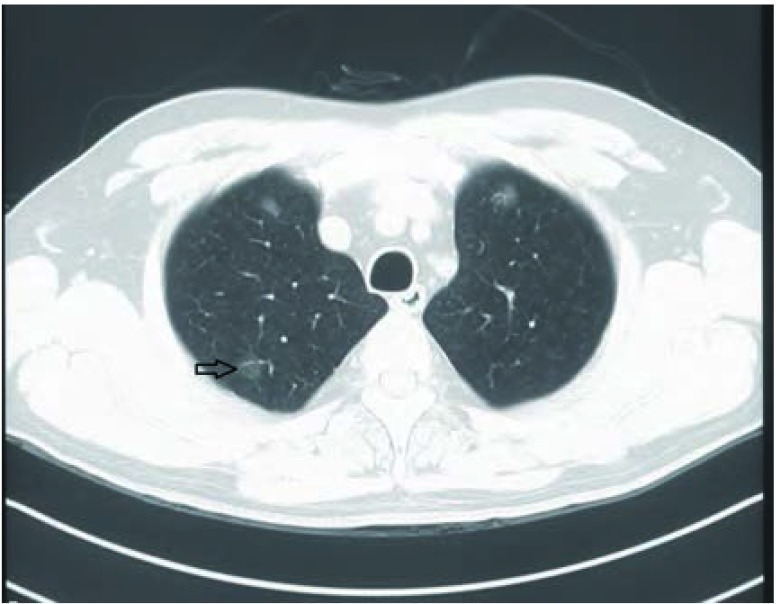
胸部CT检查。右上肺叶尖段磨玻璃样结节（箭头所指）。 CT scans of chest show the ground-glass nodule in apical segment of the superior lobe of the right lung (arrowhead).
